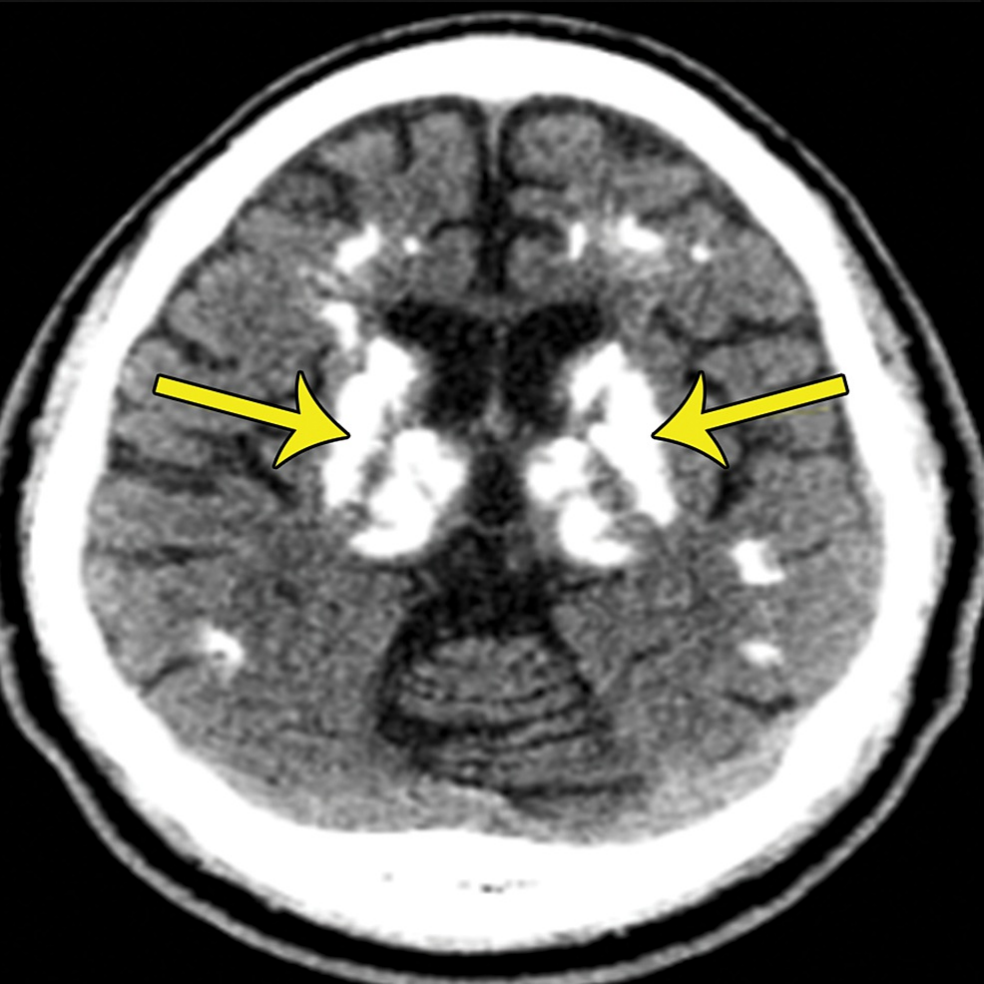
The axial non-contrast CT scan demonstrates symmetric calcification of the thalami and basal ganglia (yellow arrows) CT: computed tomography
